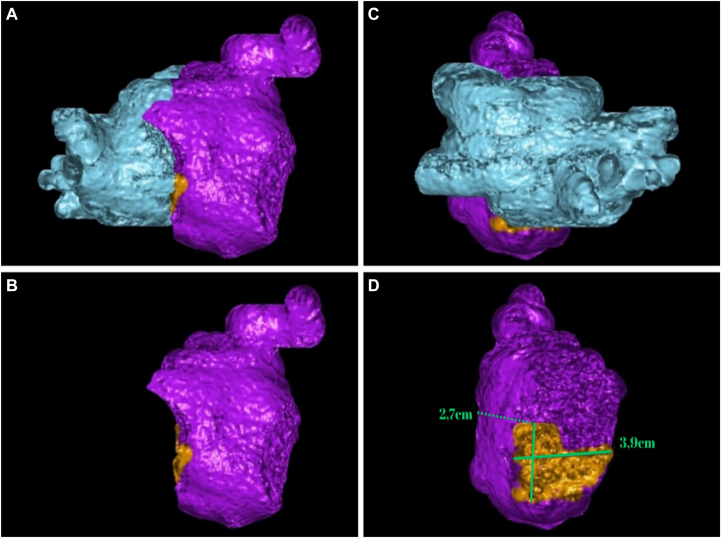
Computed tomography visualization of the LA. A–B: RAO projection of the LA with its compartments. The mitral annulus and the LAA are in the inferior-anterior compartment (*purple*) of CTS. C: PA projection of LA with 4 pulmonary veins in the posterior-superior compartment (*blue*) of CTS. D: PA projection of LA with removed posterior-superior compartment and demonstration of fibromuscular membrane (*orange*), diameter 2.7 × 3.9 cm. CTS = cor triatriatum sinister; LA = left atrium; LAA = left atrial appendage; PA = posterior-anterior; RAO = right anterior oblique.
